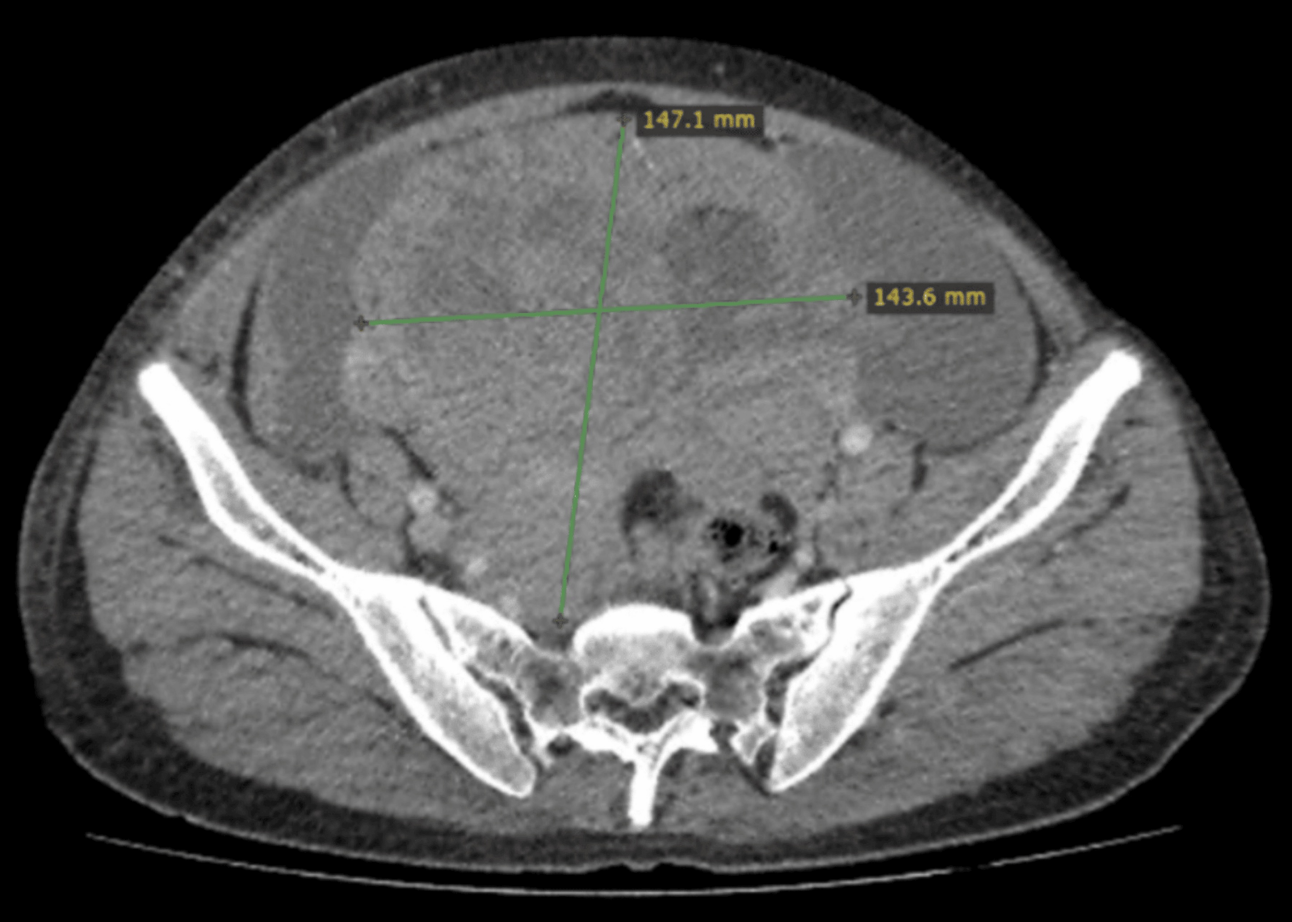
Axial image of a CT scan showing around 15×15 cm mass in the pelvis CT: computed tomography
